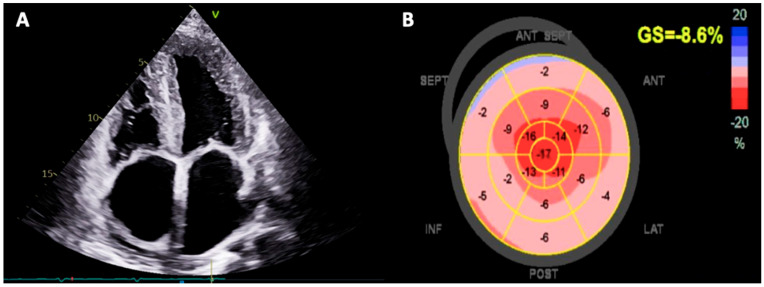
Transthoracic echocardiographic images. Panel (A) shows an apical four-chamber view with typical echocardiographic signs of cardiac amyloidosis, i.e., biventricular hypertrophy with granular sparkling pattern, severe bi-atrial enlargement and retro-atrial pericardial effusion. Panel (B) shows the global longitudinal strain (GLS) values with the peculiar apical sparing pattern (“cherry on top” pattern), obtained by two-dimensional speckle-tracking echocardiography.
